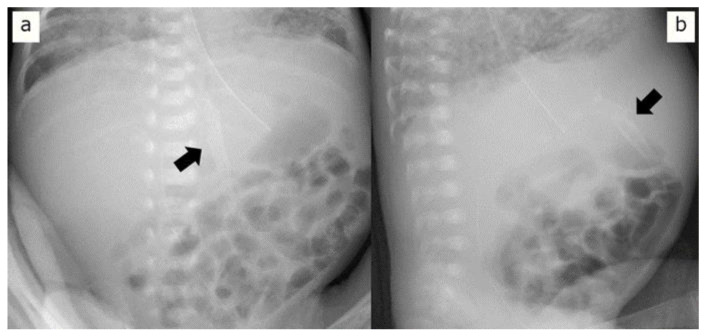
X-ray images 11 days after birth. (a) Frontal anteroposterior X-ray shows tram-track calcifications in the left upper quadrant (arrow). (b) Lateral cross-table view of the abdominal X-ray shows the calcification involving the umbilical vein (arrow).
